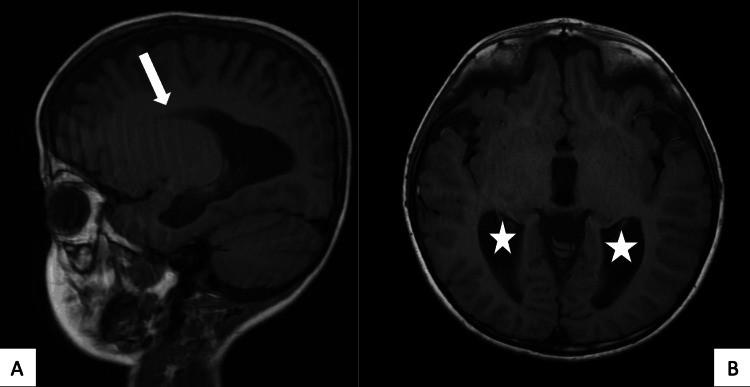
Brain magnetic resonance imaging. Panel A: Complete absence of corpus callosum (white arrow). Panel B: Dilated occipital horns and prominent trigones (white star).
